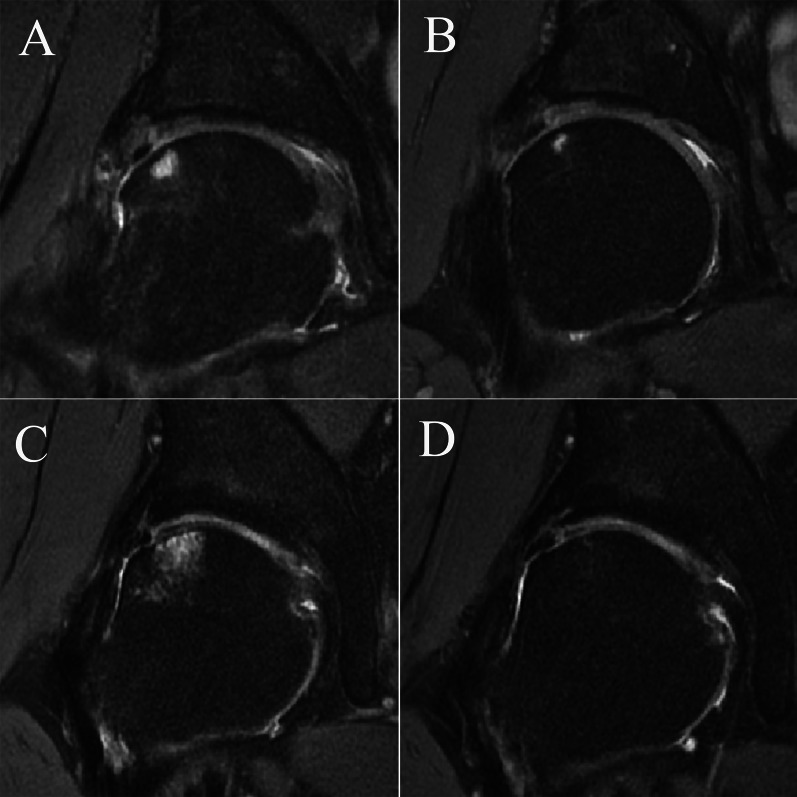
A Postoperative FHCI identified by MRI 1 year after hip arthroscopy. B The same patient underwent MRI again 6 months later, and the range of subchondral bone marrow edema of FHCI was significantly reduced. C Postoperative FHCI identified by MRI of another patient 1 year after hip arthroscopy. D Subchondral bone marrow edema of FHCI disappeared 6 months after the first MRI examination in this patient
